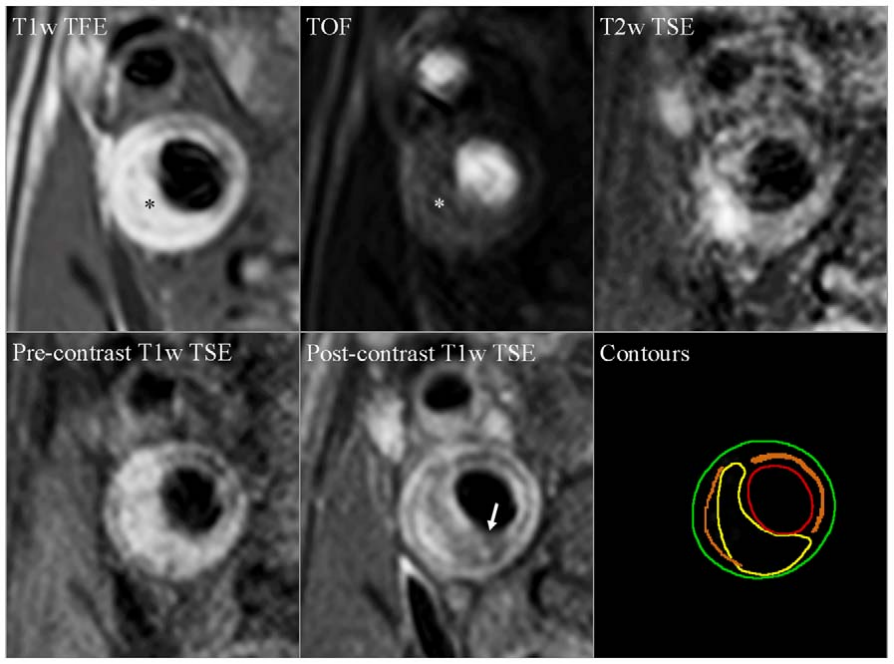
Co-registered T1-weighted TFE, TOF, T2-weighted TSE, pre- and post-contrast T1-weighted TSE images of a transverse section of a plaque in the internal carotid artery. The right bottom panel displays the plaque components: red = lumen; green = outer vessel wall; yellow = LRNC; orange = calcifications; remaining vessel wall area = fibrous tissue. IPH was scored as being present (asterisk in T1-weighted TFE and TOF images) and the FC was designated as thin and/or ruptured (arrow in post-contrast T1-weighted TSE image).
